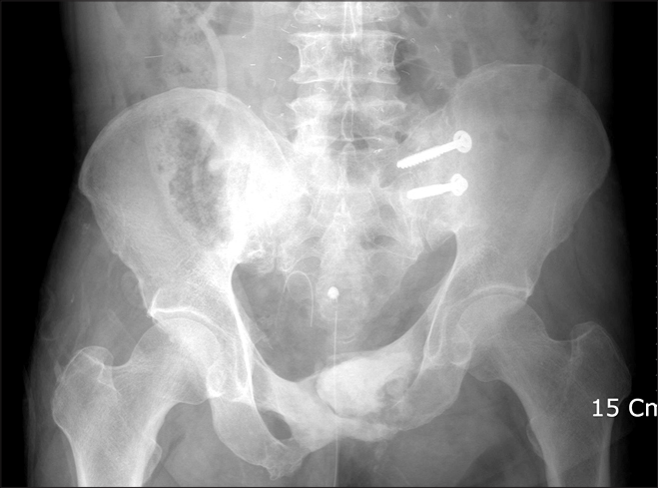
Postoperative anteroposterior radiograph of the pelvis.
